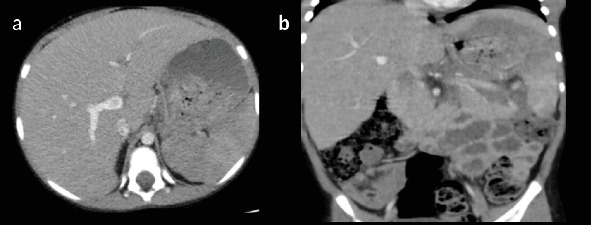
Postchemotherapy axial CT images. (a) Homogeneous liver parenchymal enhancement. (b) Normalization of splenic size to 12.7 cm.
